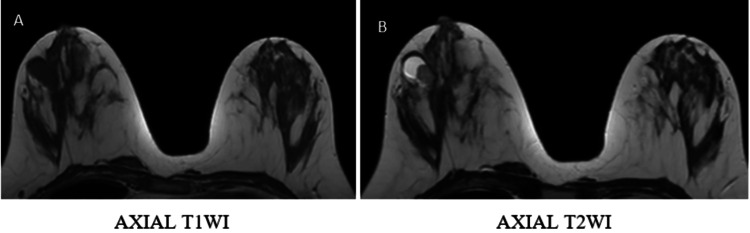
BI-RADS 4 lesion shows a solid-cystic mass in the outer quadrant of the right breast. The lesion appears isointense on T1WI and demonstrates a hyperintense cystic component with intermediate to mildly hyperintense signal in the solid component on T2WI (A, B) BI-RADS: Breast Imaging Reporting and Data System; T1WI: T1-weighted image; T2WI: T2-weighted image
